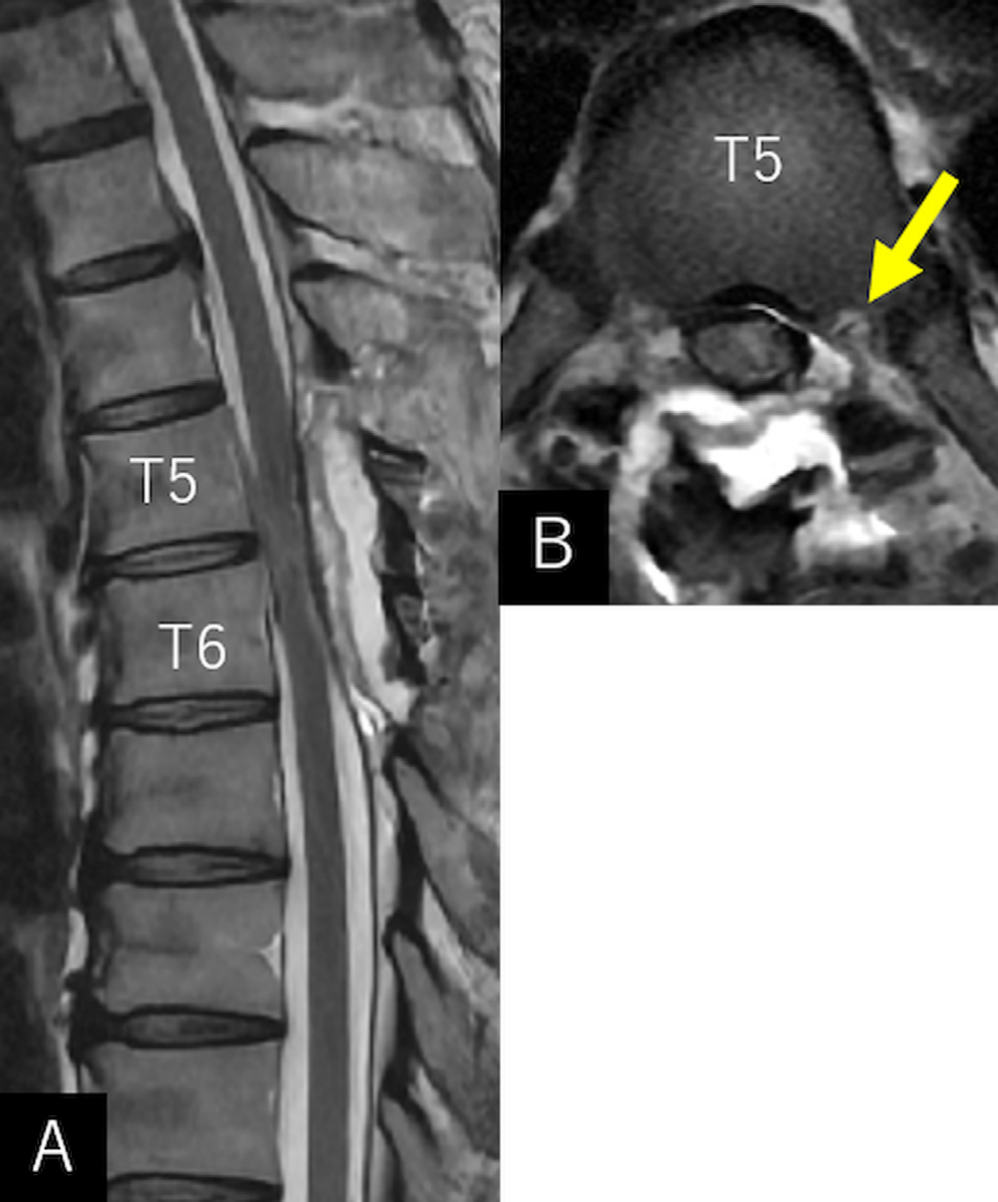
Sagittal (A) and axial (B) T2-weighted magnetic resonance imaging performed on the postoperative day 7 showing a restoration of herniated cord with complete separation between the dura sac and erosion in the lower T5 vertebra (*arrow*).
